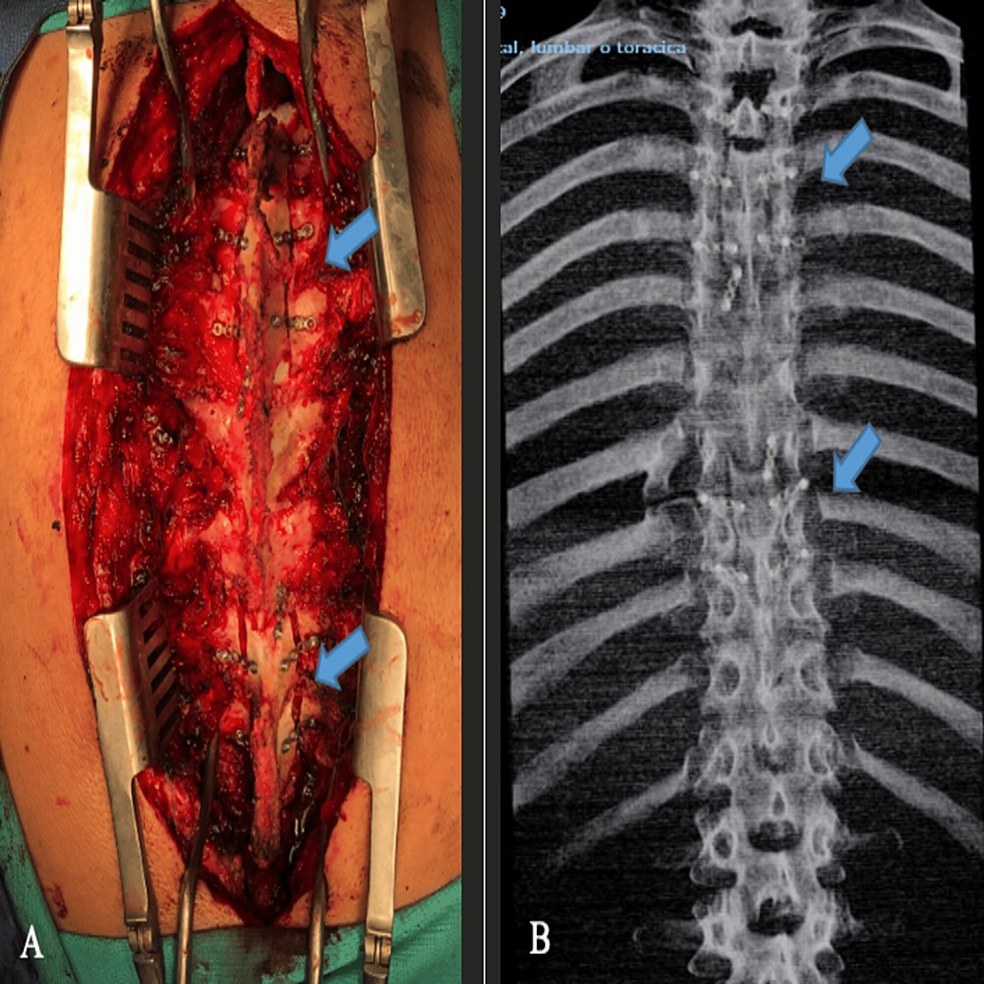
Reconstruction of the approach and postsurgical image (A) Laminoplasty with titanium plate and screws (blue arrows). (B) A simple coronal radiograph showing laminoplasties (blue arrows).
